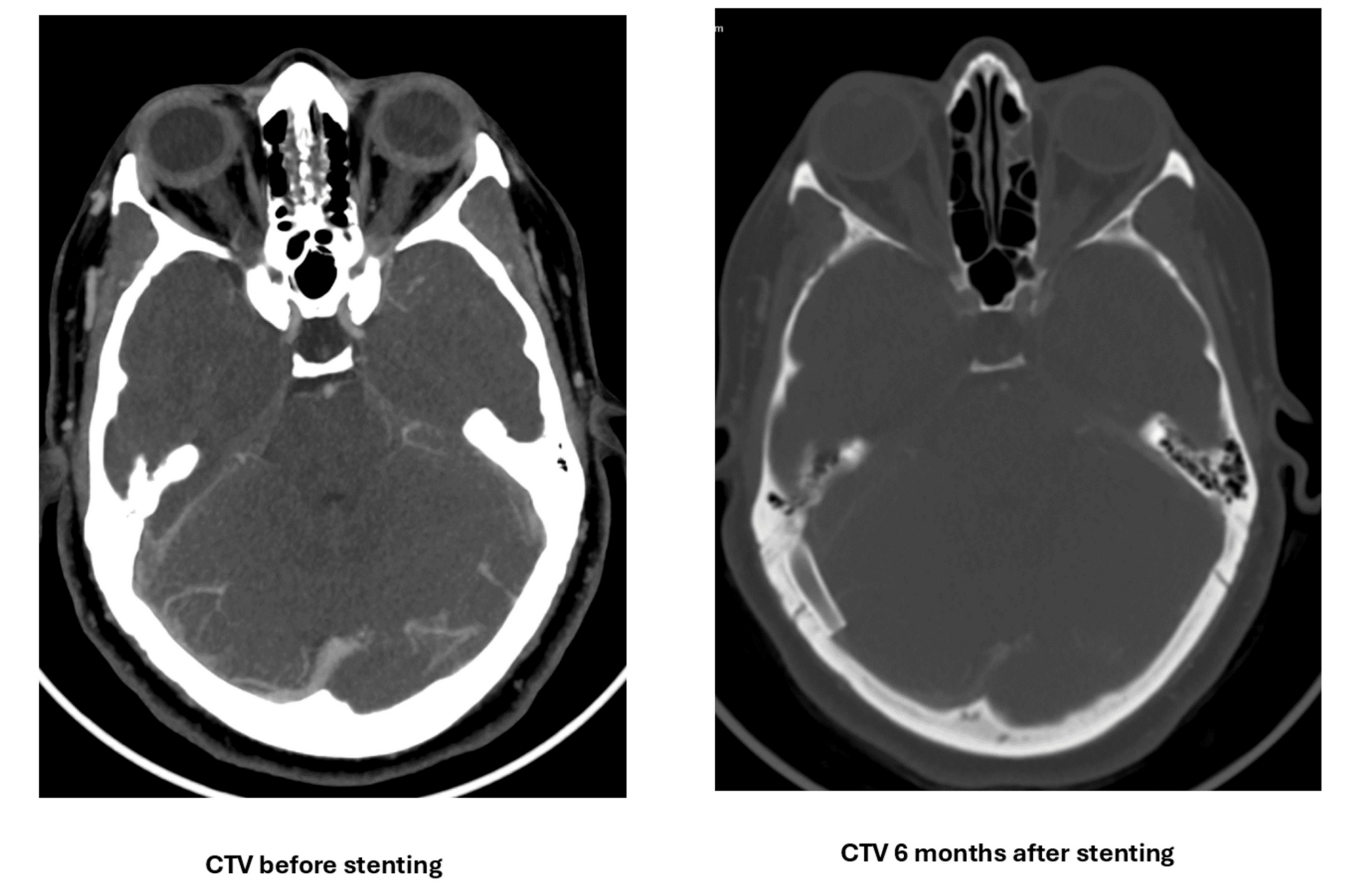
CTV before and after stenting showing good patency of the stent in the right transverse sinus CTV: computed tomographic venography
